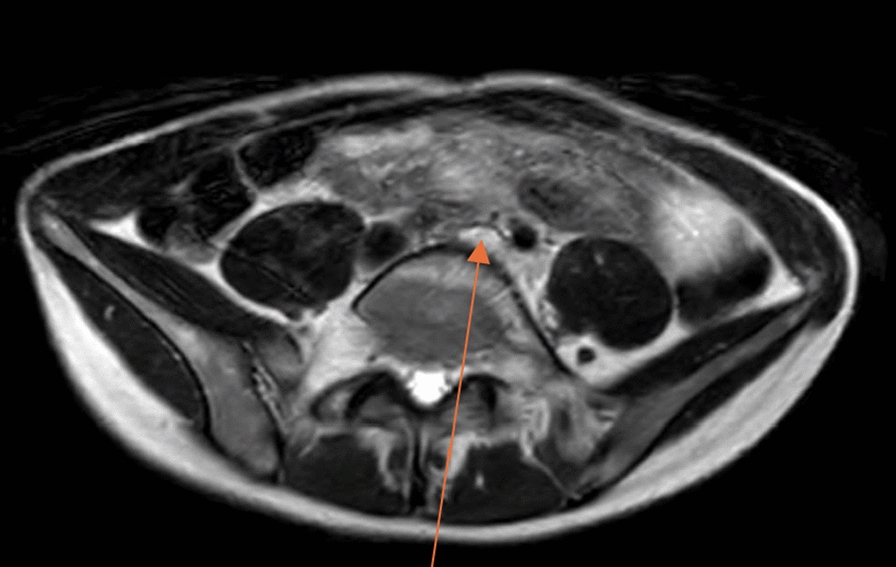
T2 weighted MRI with arrow pointing to thrombus in left common iliac vein where it is compressed
